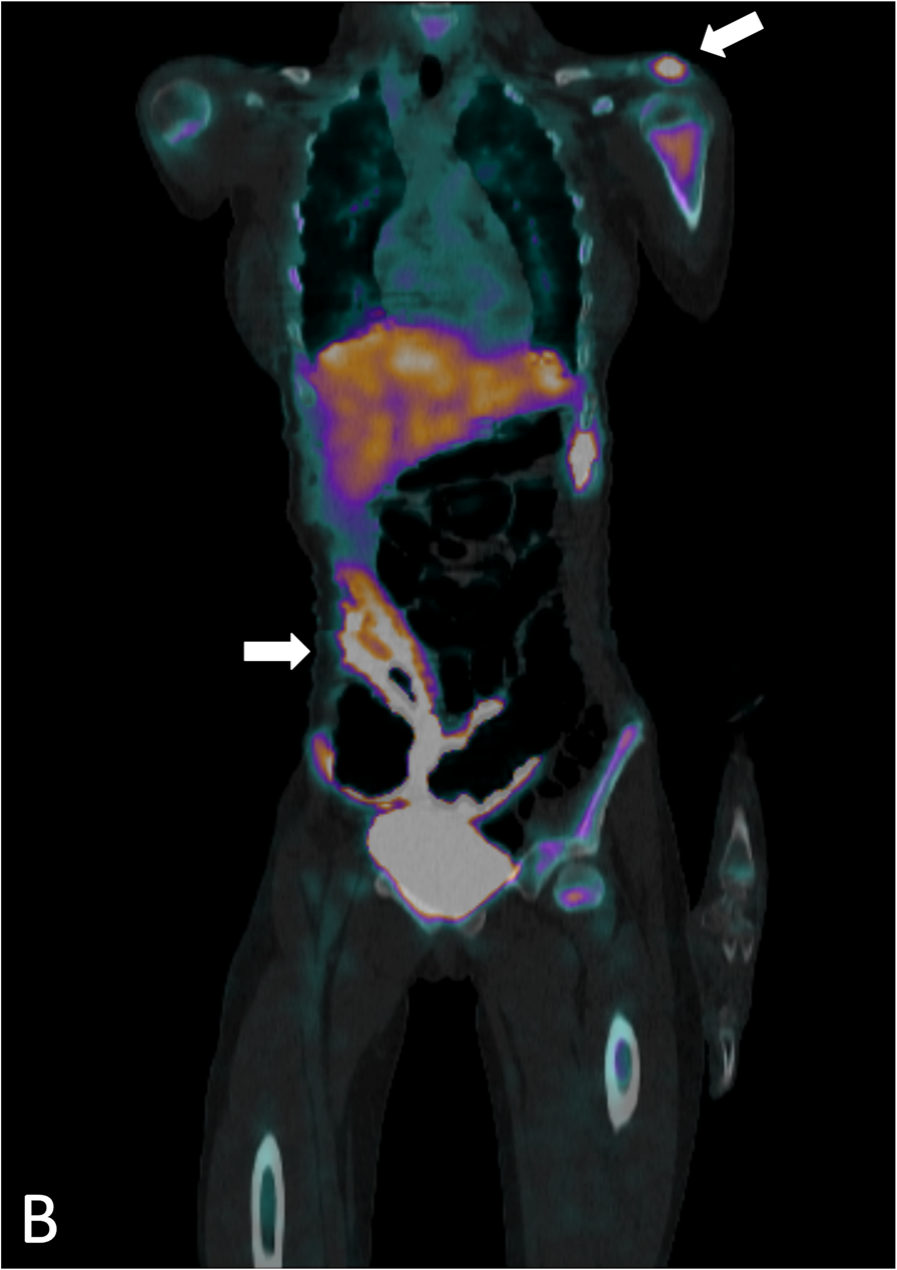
Single-photon emission computerized tomography (SPECT) scan demonstrates lytic changes at the left acromial apophysis and colon (white arrows)
